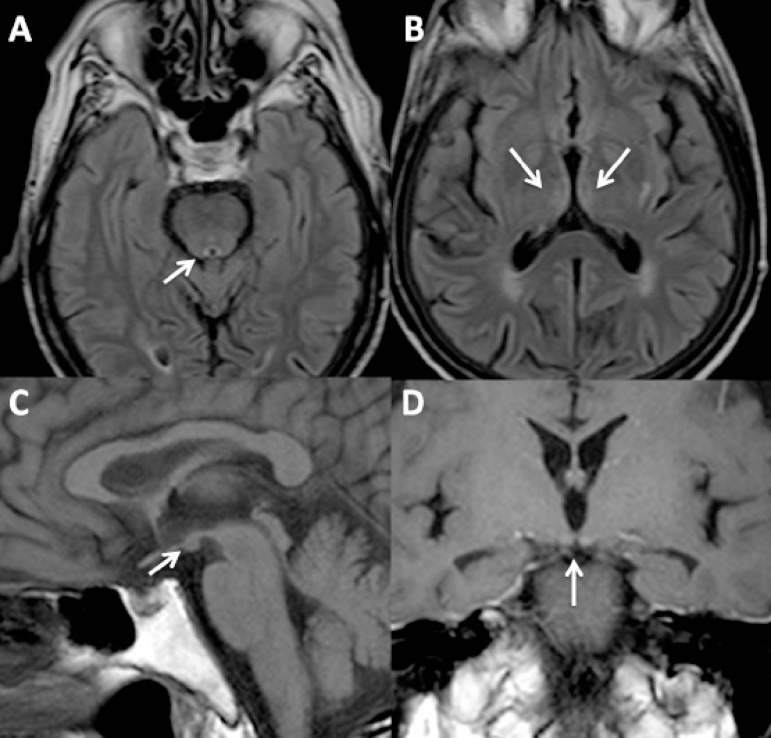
[A and B] FLAIR images depicting hyperintensities in the periaqueductal
